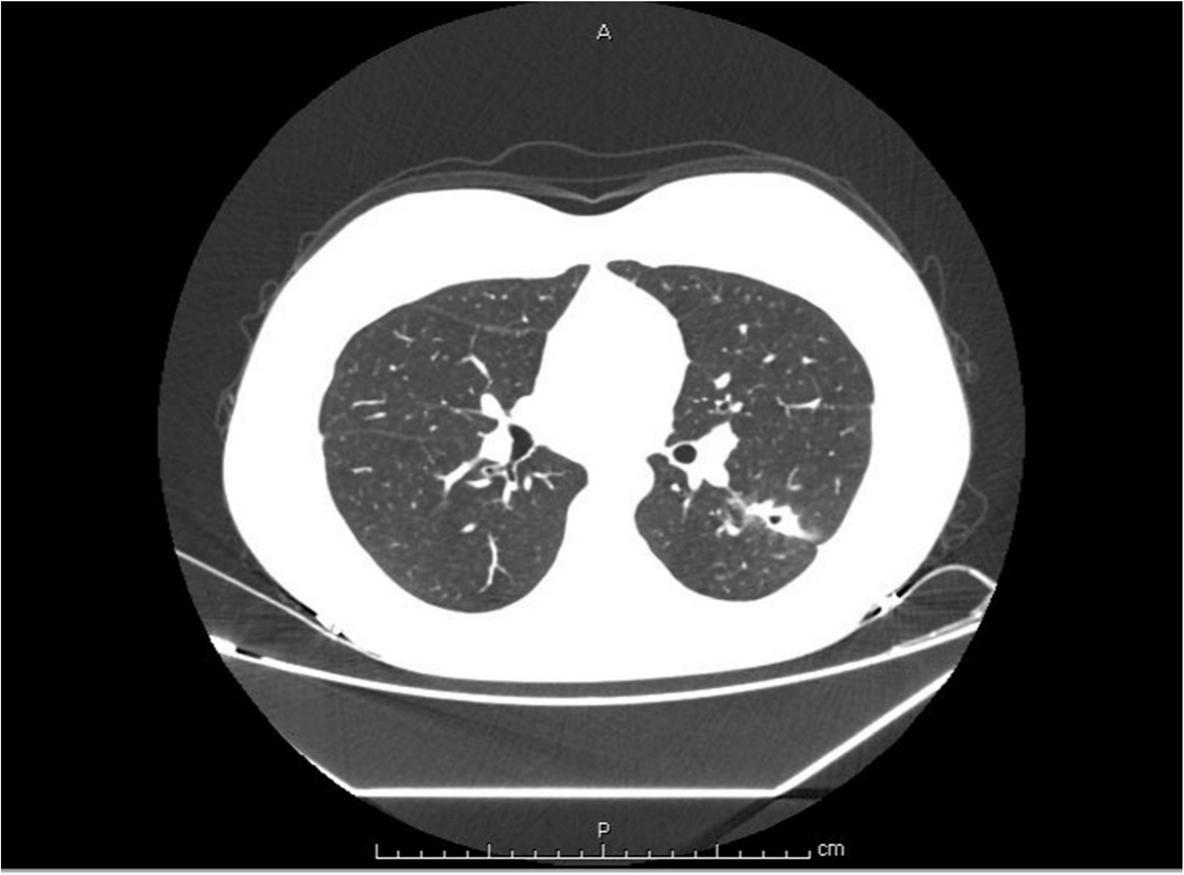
Multiple cavitary lesions and nodules in the lower left lobe were identified on chest computed tomography
